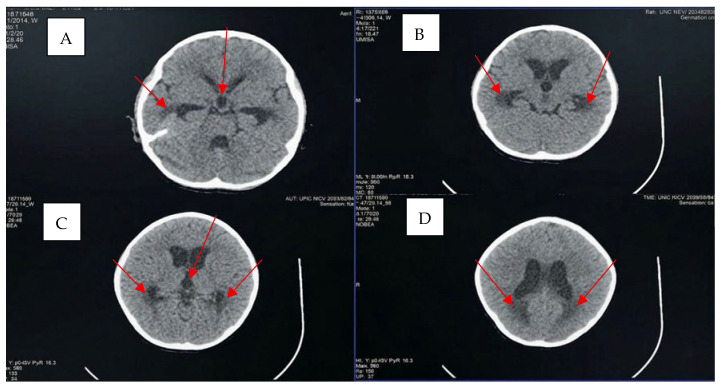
Non-contrast axial head computed tomography (CT). Red arrows indicate the identified pathological abnormalities, specifically the heterogeneous hypodense lesions and focal ventricular dilatations. (A) Bilateral hypodense regions with heterogeneous attenuation and irregular margins are identified lateral to the floor of the anterior temporal horns. An additional heterogeneous hypodensity with irregular margins is noted along the anteromedial floor of the left frontal horn. Focal saccular dilatation of the posterior aspect of the third ventricle is also present. (B) Bilateral hypodense regions are noted lateral to the bodies of the temporal horns; the margins of these areas conform to the convexity of the adjacent ventricular structures. Saccular dilatation of the mid-portion of the third ventricle is evident. (C) Bilateral heterogeneous hypodensities with irregular margins are located lateral to the ventricular atria, accompanied by focal saccular dilatation of the anterior aspect of the third ventricle. (D) Bilateral heterogeneous hypodensities with irregular margins are identified posterior to the central portions of the frontal horns.
